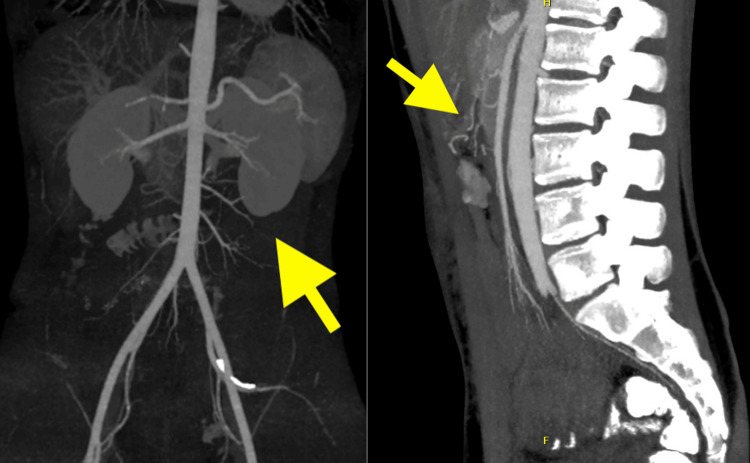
CT angiography revealed a delicate left colic artery branching toward the splenic flexure. CT: computed tomography
